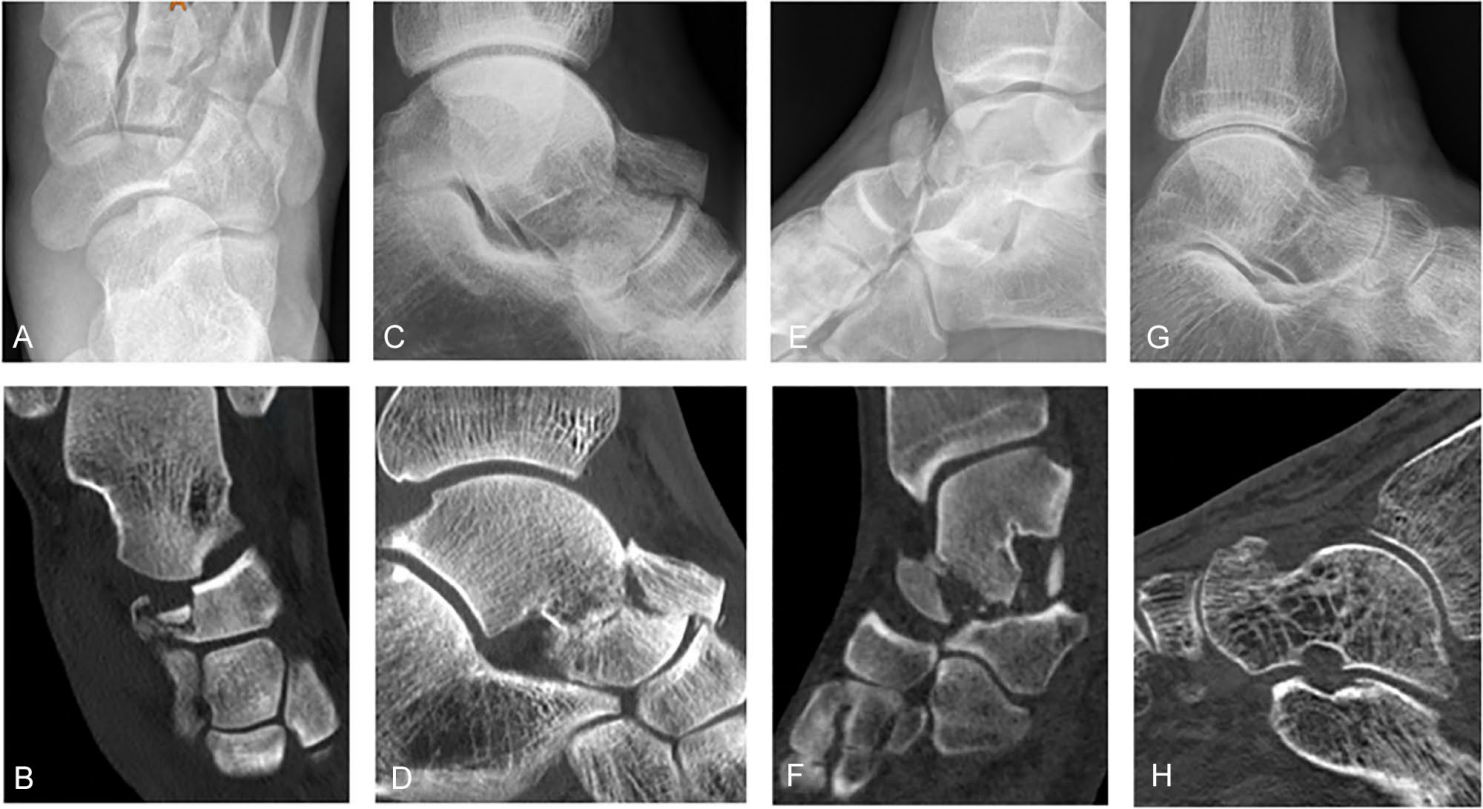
Four types of talar head fractures identified. Different fracture types of the talar head as shown in conventional radiographs (top row) and computed tomography scans (bottom row). (A, B) Impaction fracture of the talus head. (C, D) Complete transversal fracture of the talus head and fracture of the talus neck. (E, F) Medial shear fracture of the talus head, best seen in the sagittal plane. (G, H) Avulsion fracture of the talus head, first presented in our hospital at 4 months after trauma. Fracture was initially missed.
